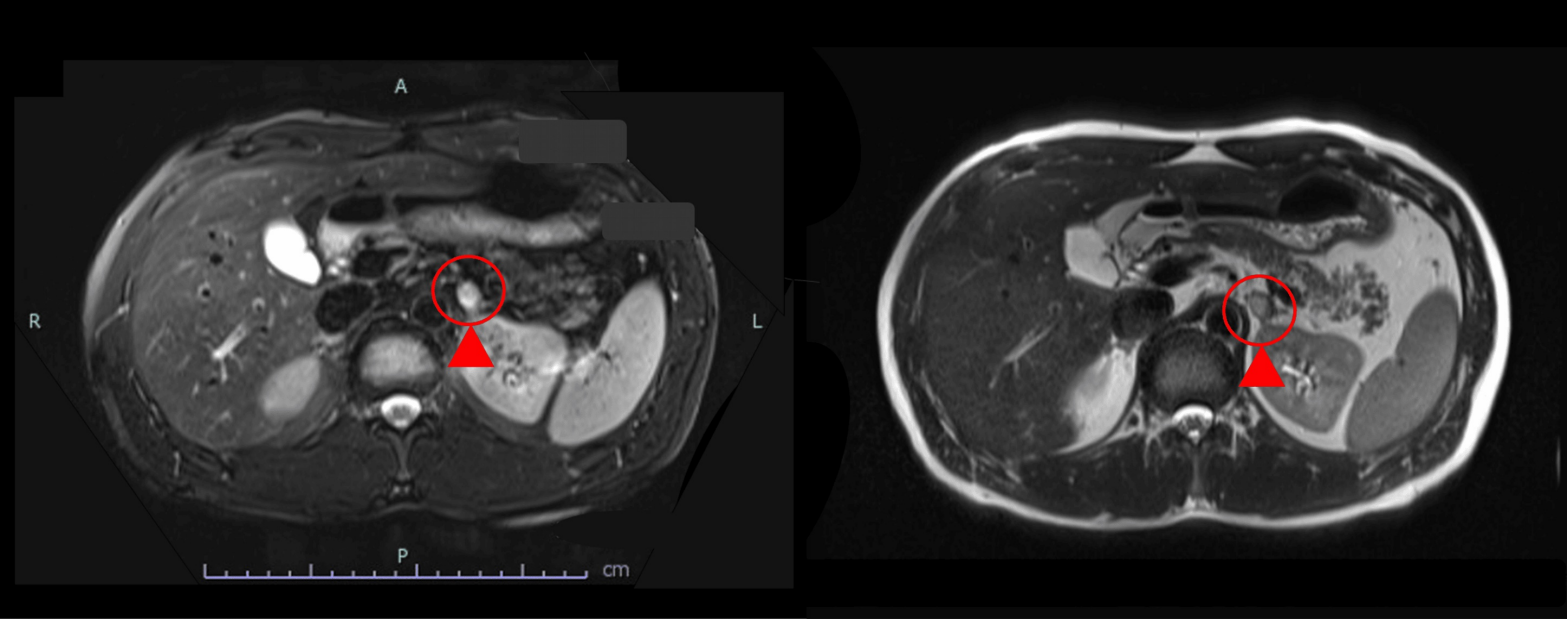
Abdominal MRI findings The findings show an oval mass in front of the left kidney (▲).
